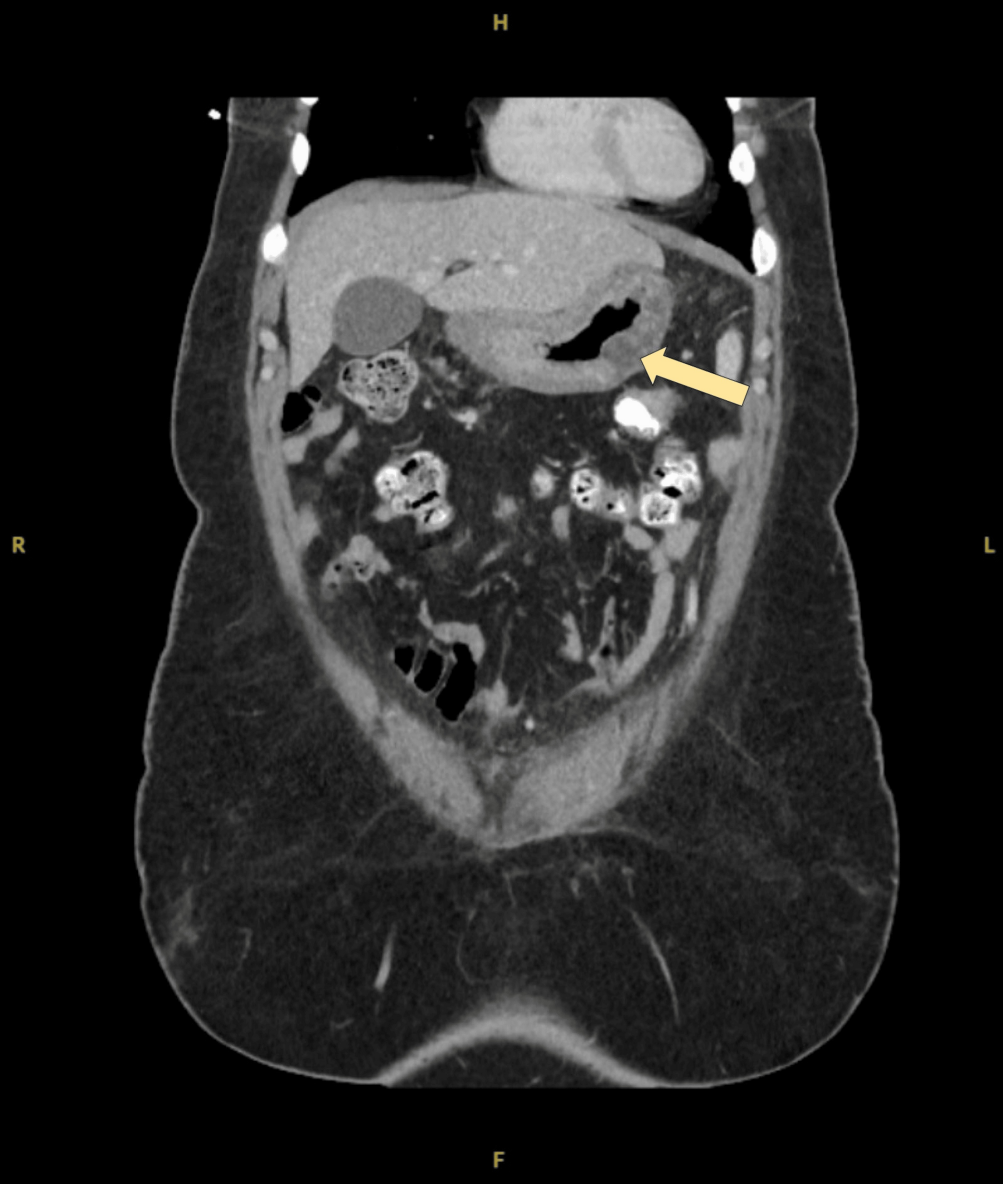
CT of the abdomen and pelvis (coronal view) demonstrating diffuse gastric mucosa thickening and a hypodense mass (arrow) within the greater curvature of the stomach CT: computed tomography
